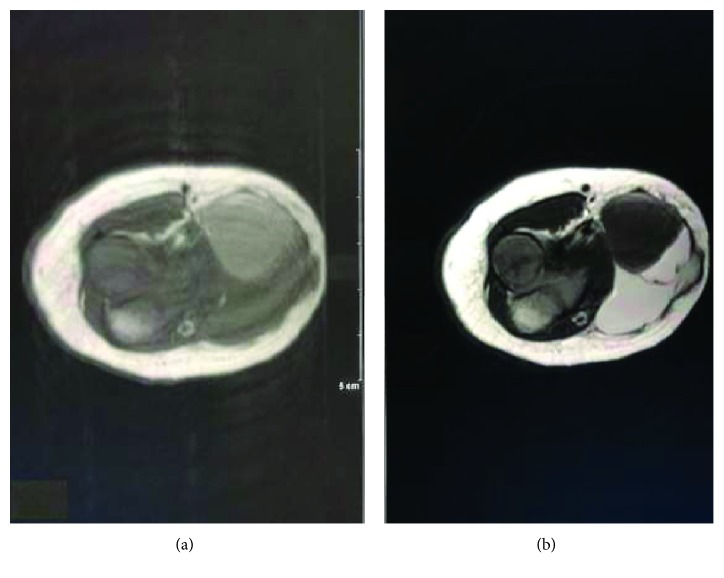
MRI of the right elbow axial T1-W1 (a) and T2-W1 (b) intramass 1.8 × 1.8 × 1.5 cm area with low T2 and high T1 signal intensity.
